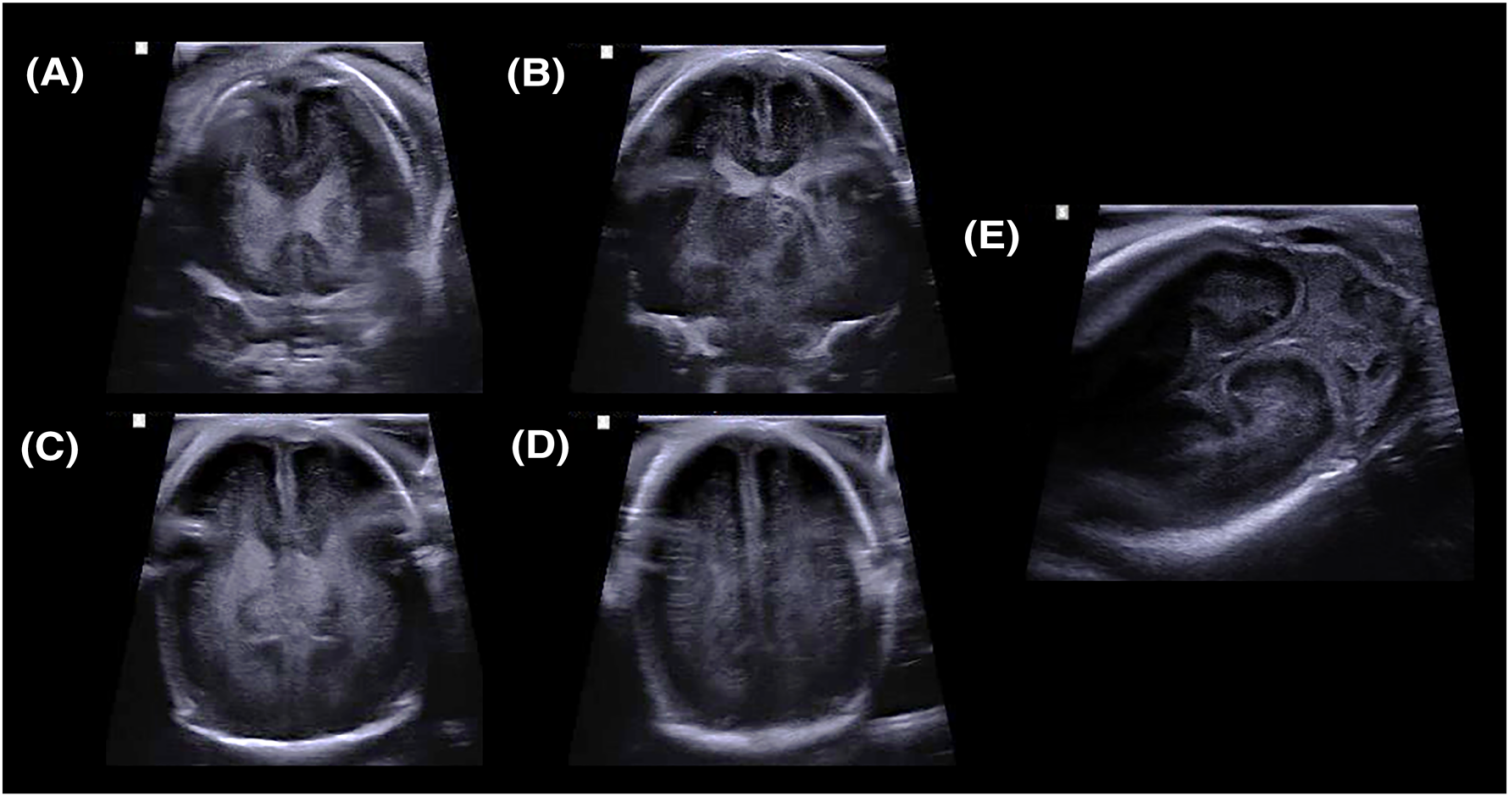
Example of a diagnostic quality post‐mortem ultrasound image of the brain in a 17‐week gestational aged fetus, obtained in a water bath. Coronal ultrasound images through A, the frontal horns of the lateral ventricles, B, the body of the lateral ventricles, C, the trigone of the lateral ventricles, and D, the occipital lobes respectively. Image (E) demonstrates image acquisition via the mastoid fontanelle, depicting the cerebellar hemispheres in coronal section [Colour figure can be viewed at http://wileyonlinelibrary.com]
